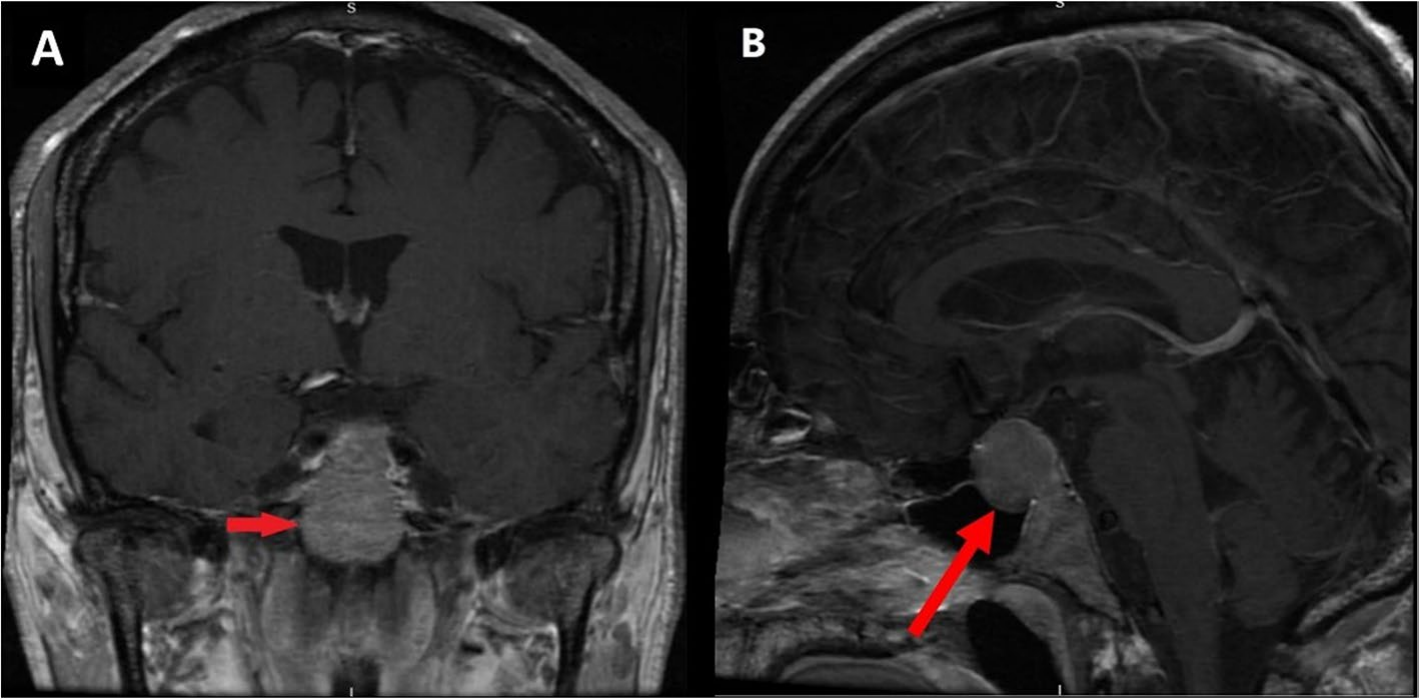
Initial MRI pituitary with contrast. A T1 coronal MRI showing pituitary macroadenoma (2 × 1.9 × 1.5 cm). B T1 sagittal MRI showing pituitary macroadenoma
